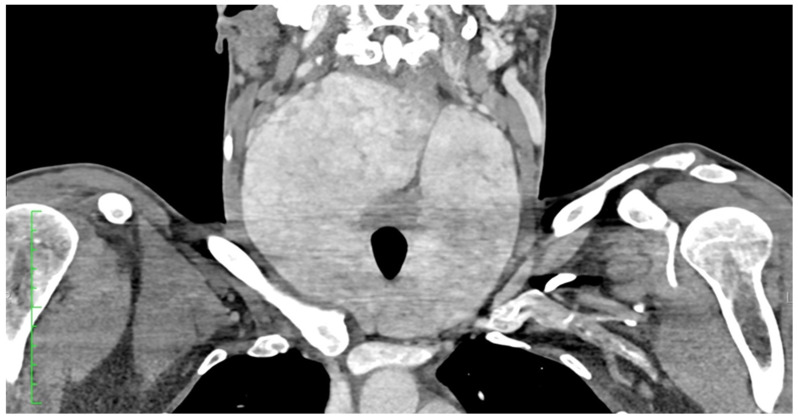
Neck CT. Axial. The contours of the thyroid gland are relatively smooth, causing displacement and compression of the surrounding structures: (1) bilateral displacement of the carotid vessels; (2) medial and rightward displacement of the oesophagus; (3) compression and slight rightward deviation of the trachea; (4) bilateral lateral displacement of the sternocleidomastoid muscles.
